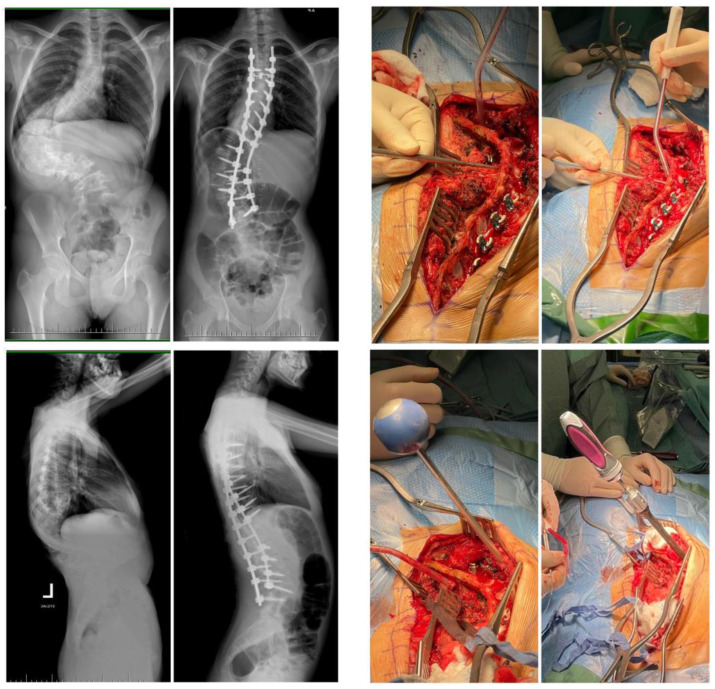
Pre- and postoperative radiographs of a 14-year-old girl with severe and neglected adolescent idiopathic scoliosis, treated surgically via posterior approach with free-hand segmental pedicle screw fixation and fusion; intraoperative pictures show great difficulties and very risky starting point for screw insertion due to severe rotation and angulation of the lumbar spine.
